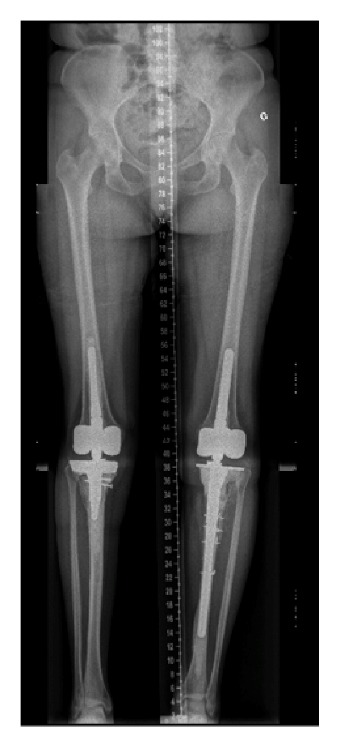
Follow-up X-rays, three months after osteosynthesis of the left tibial periprosthetic fracture.
